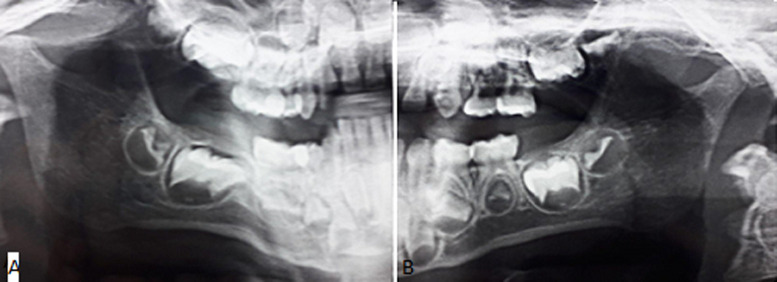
panoramic radiograph showing the absence of dental or alveolar bone anomalies A) right side; B) left side
